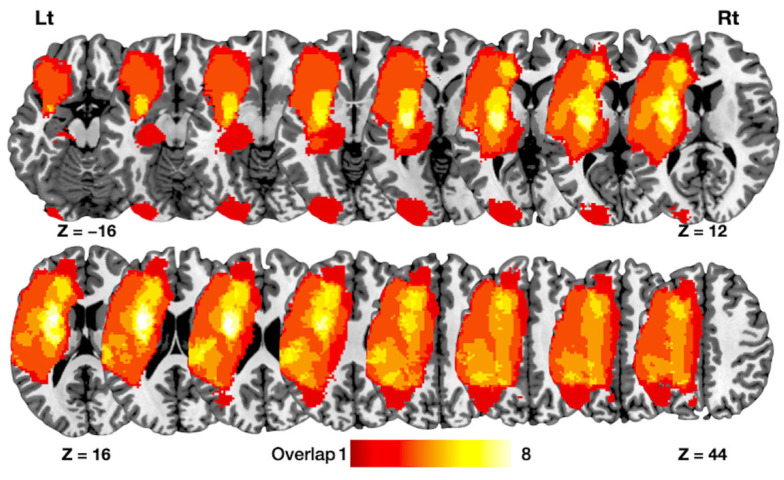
Lesion overlapping maps. Overlay map showing the lesion location in each of the patients 20 included in the study. A T1-weighted map was used to demarcate the lesion corresponding to each patient. The color scale indicates the degree of lesion overlap between patients, with the maximum overlay corresponding to eight patients. Rt = right hemisphere. Lt = left hemisphere. z = *z*-axis in Montreal Neurological Institute space.
